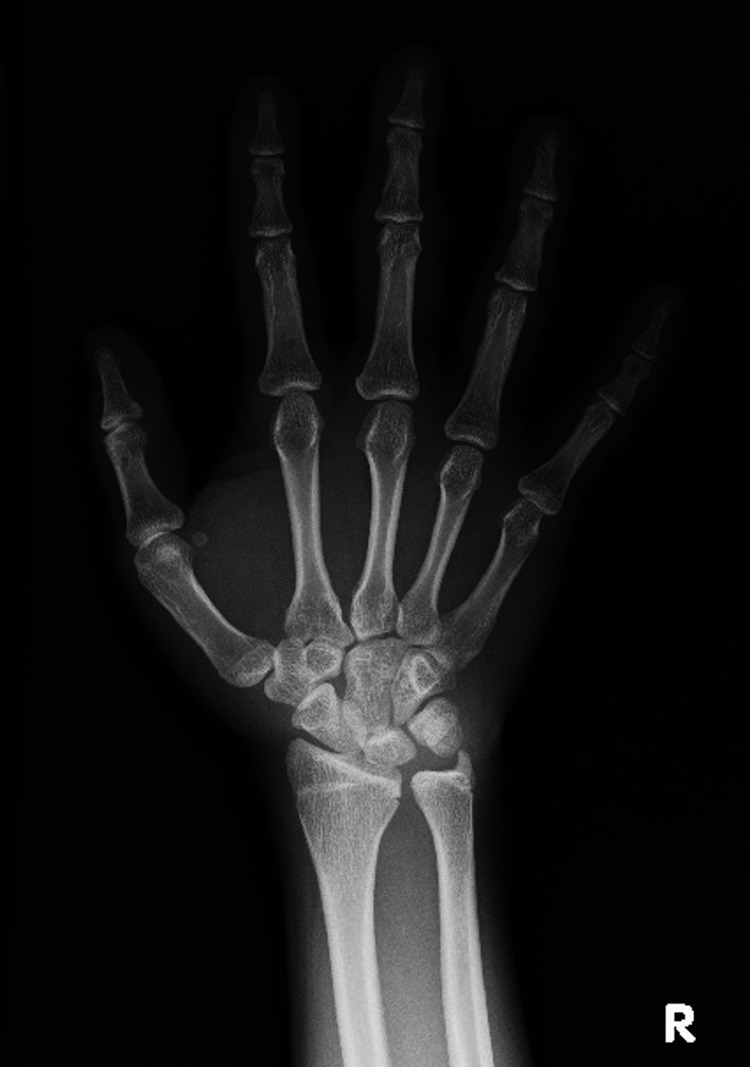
Plain radiograph Plain radiographs of the hands showing no bone destruction or joint space narrowing.
